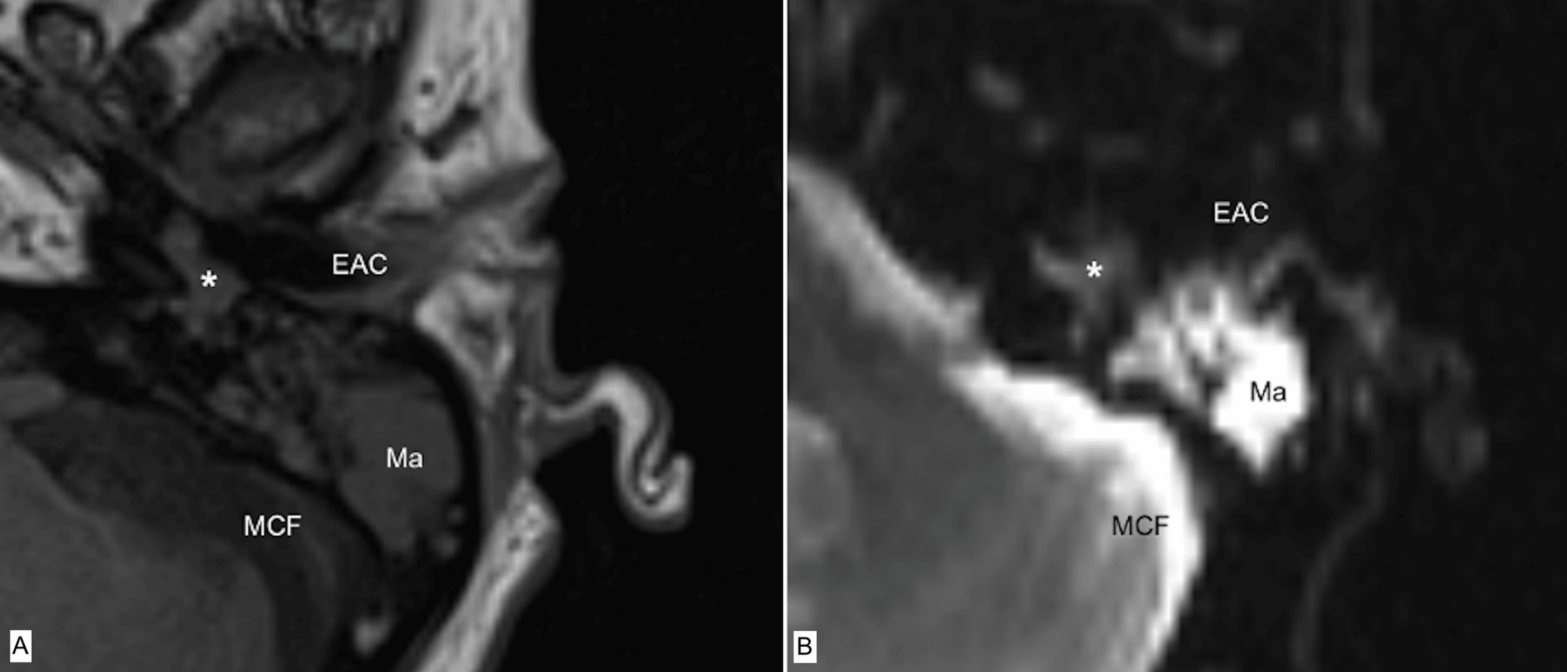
MRI of the left temporal bone showing a mass in the antrum (asterisk), later confirmed as MeNET A: T1-sequence, B: Diffusion-weighted sequence, Ma: Mastoid, EAC: External auditory canal, * indicating MeNET
